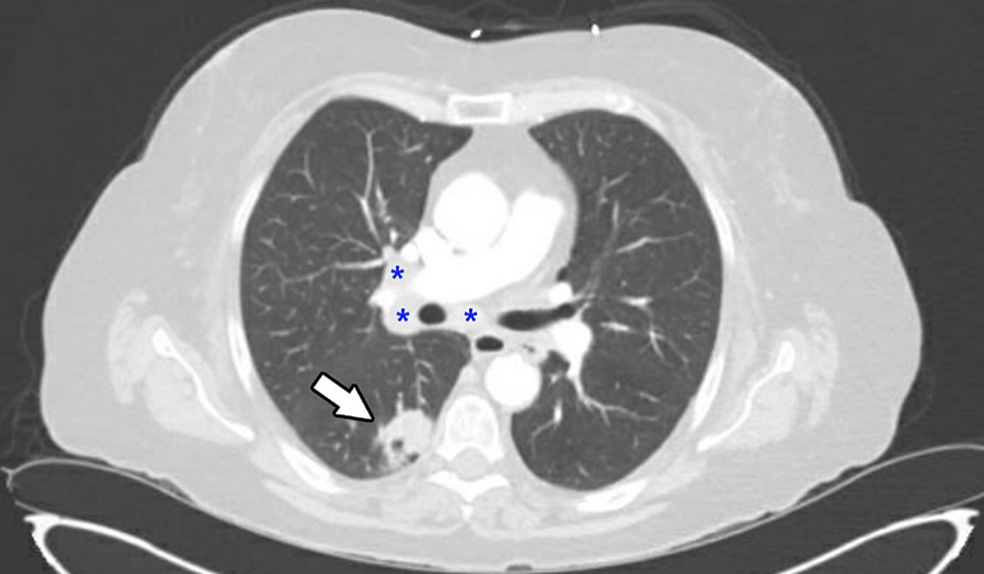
Computed Tomography of the Chest with Intravenous Contrast White arrow: Adenocarcinoma of the superior segment of the right lower lobe Blue asterisks: Right hilar adenopathy and subcarinal lymph node
